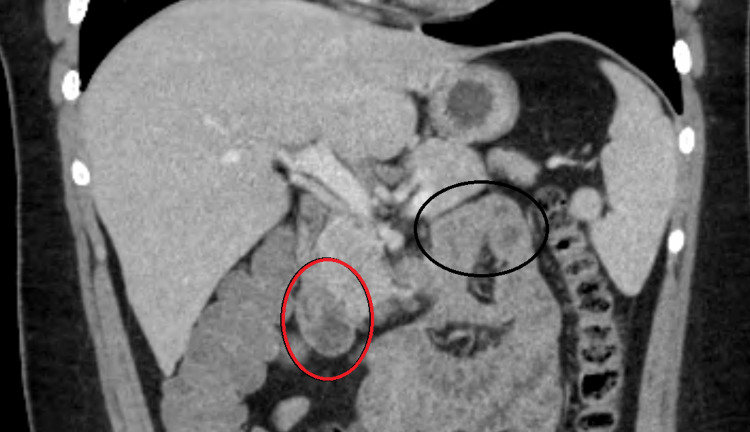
Abdominal CT scan (coronal) showing a nearly 180-degree directional relationship between the transition from the second to third segments of the duodenum (red oval) and the location of the duodenojejunal flexure (suspensory ligament, or ligament of Treitz) (black oval).
